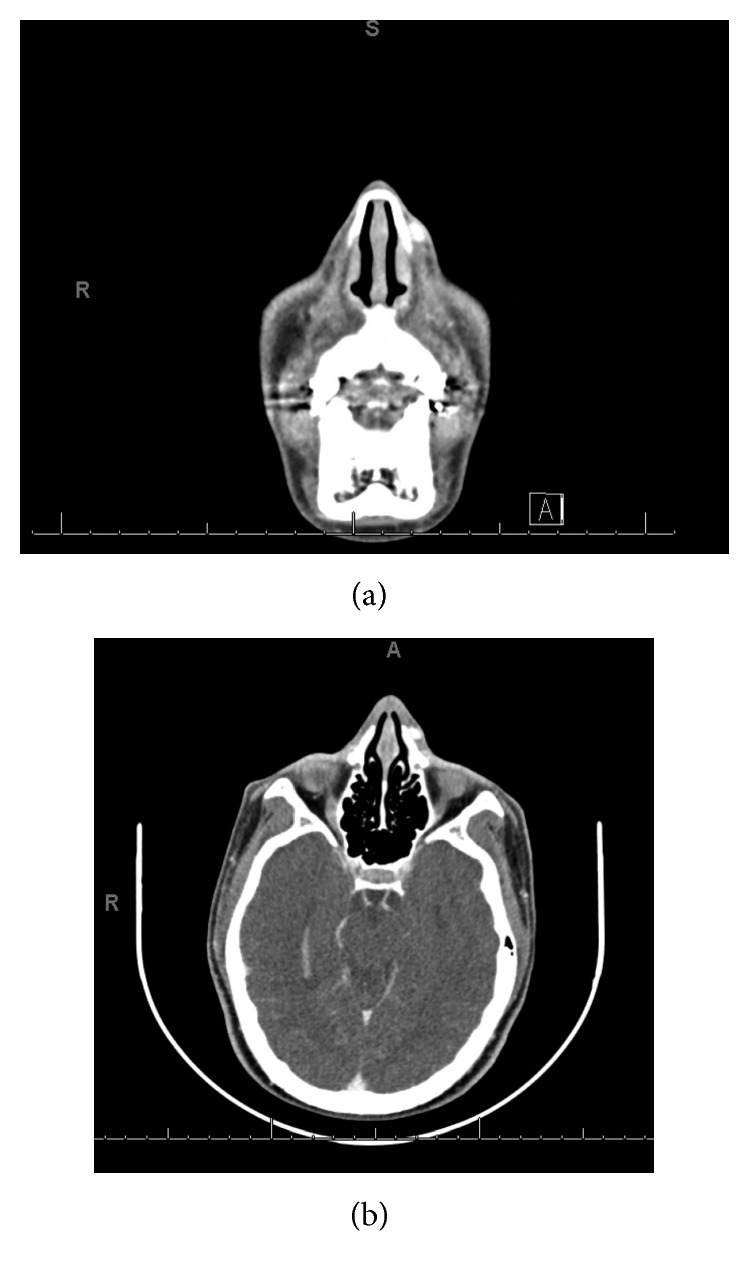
Axial contrast-enhanced CT images showing a well-demarcated, hyperdense, and nonlipomatous mass involving soft tissue of the left lateral nasal wall.
